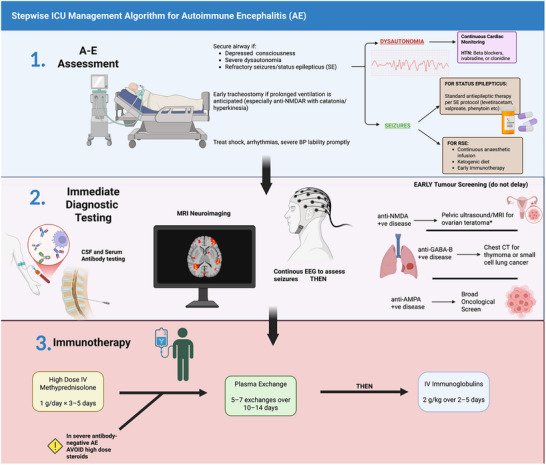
Stepwise management of AE in ICU. *Although testicular teratomas are much less common in males, it is also recognized that ultrasound/MRI imaging is required for the screening of all male AE patients. Figure 4 was created in BioRender. Veenith, T. (2025) https://BioRender.com/60wwnht.
